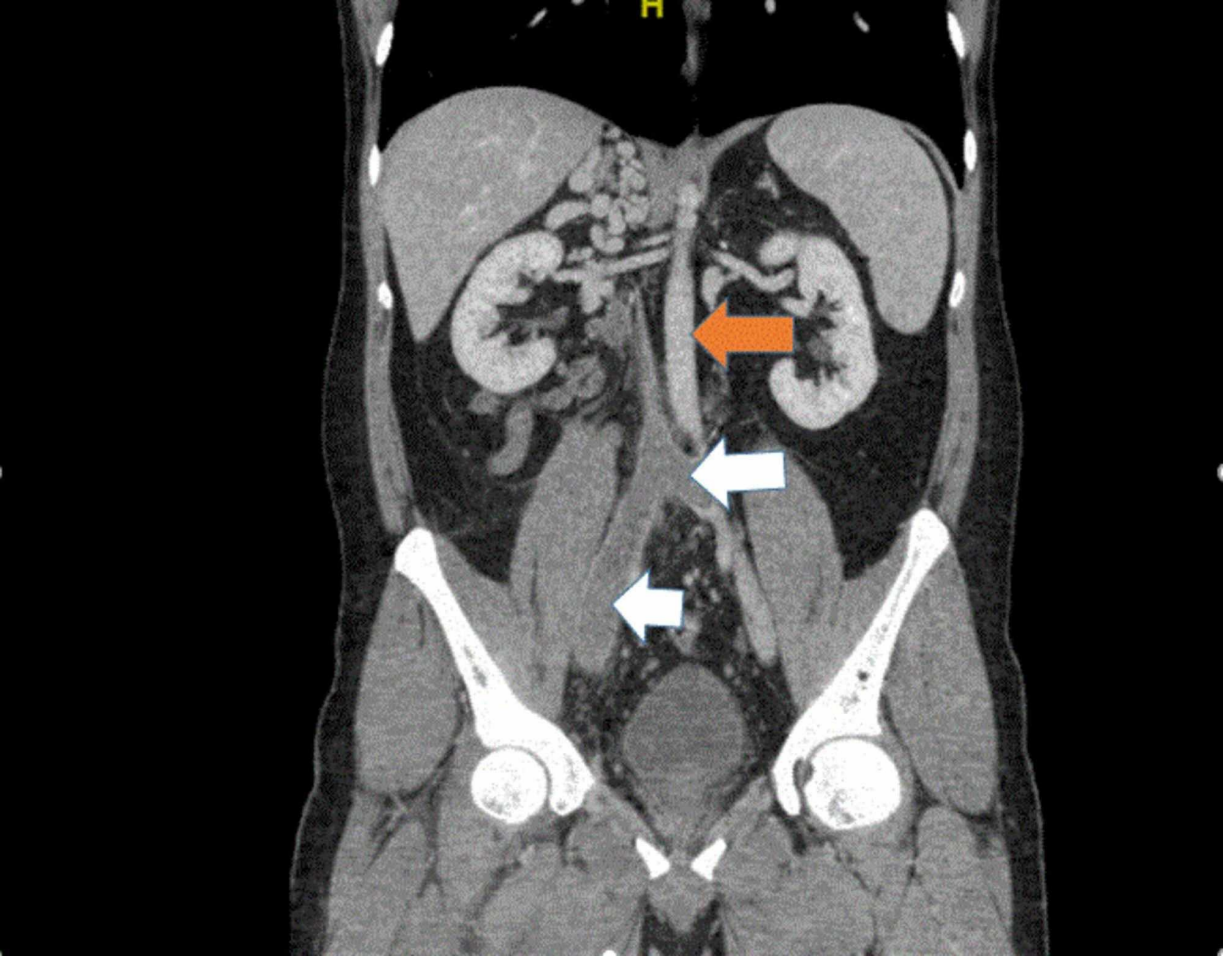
Computed tomography of the abdomen and pelvis with IV contrast, in coronal view, shows extensive right and left common iliac vein thrombosis (white arrow) and normal aorta (orange arrow)
